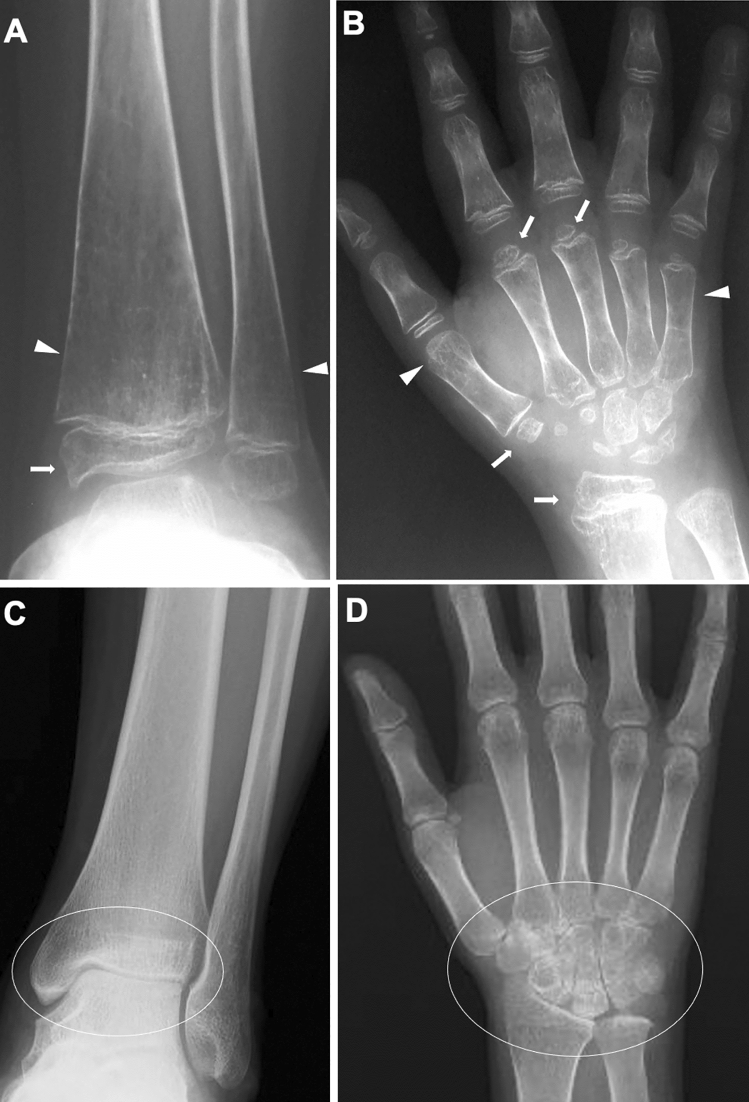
Different late sequelae between systemic JIA and polyarticular JIA. A–B. A 10-year-old boy with systemic JIA. Frontal radiographs of the left lower leg (A) and the right hand (B) show severe osteopenia, abnormal epiphyseal ossification (arrows, A, B), and metaphyseal broadening (arrowheads, A, B), but relatively spared joint spaces. C–D. A 16-year-old girl with polyarticular JIA. Frontal radiographs of the left lower leg (C) and the right hand (D) demonstrate striking joint space narrowing and minimal or mild subchondral bone irregularities (circles). Courtesy of Professor Yutaka Inaba, Department of Orthopedics, Yokohama City University Hospital
